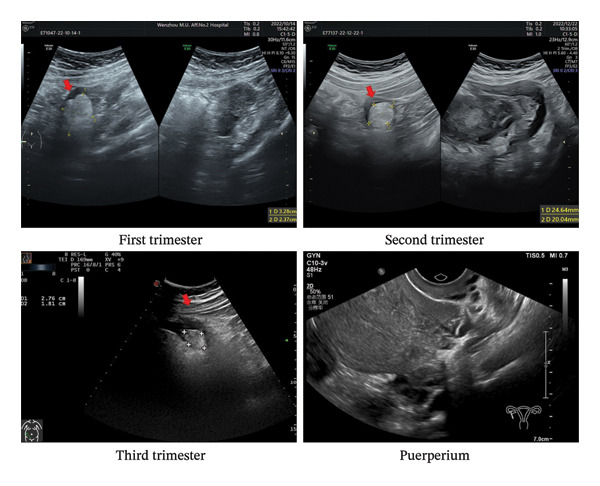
Ultrasound images of a single pregnant woman’s ovarian mass at first trimester, second trimester, third trimester, and puerperium. *Arrows* indicated the location of ovarian mass.
